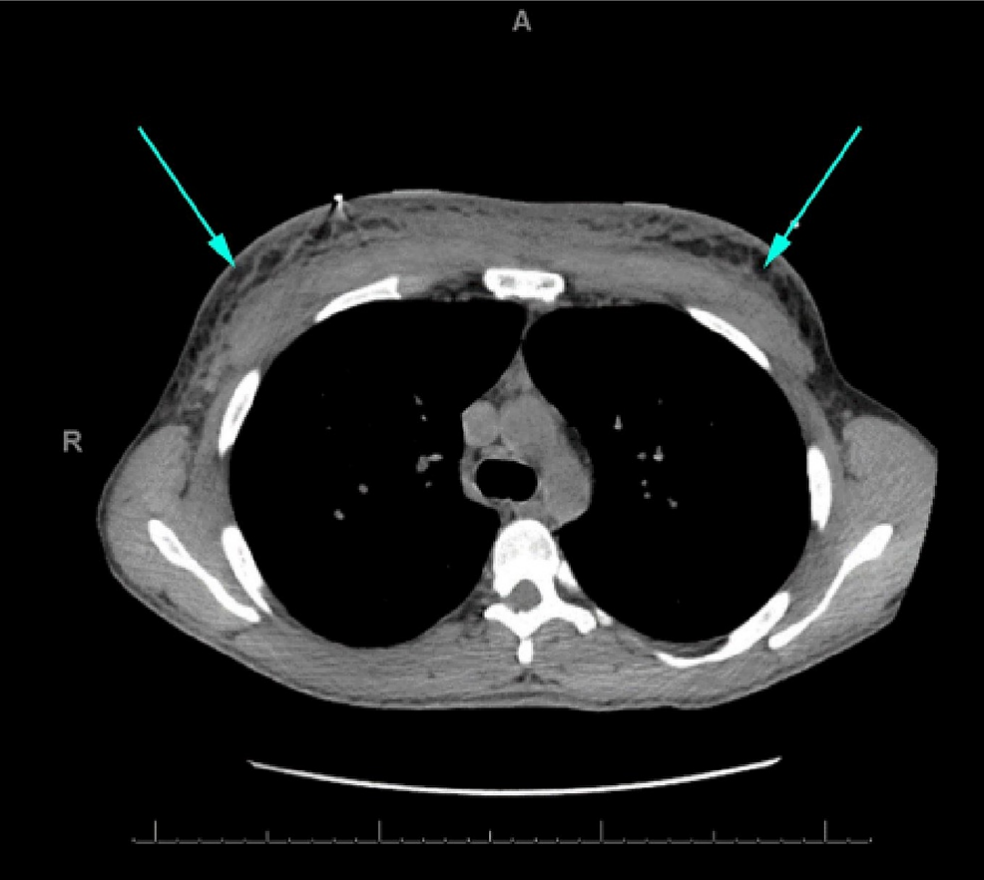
Computed tomography of the chest on admission without contrast. Axial view showing significant diffuse body wall edema with reticulation of subcutaneous tissue (arrows).
